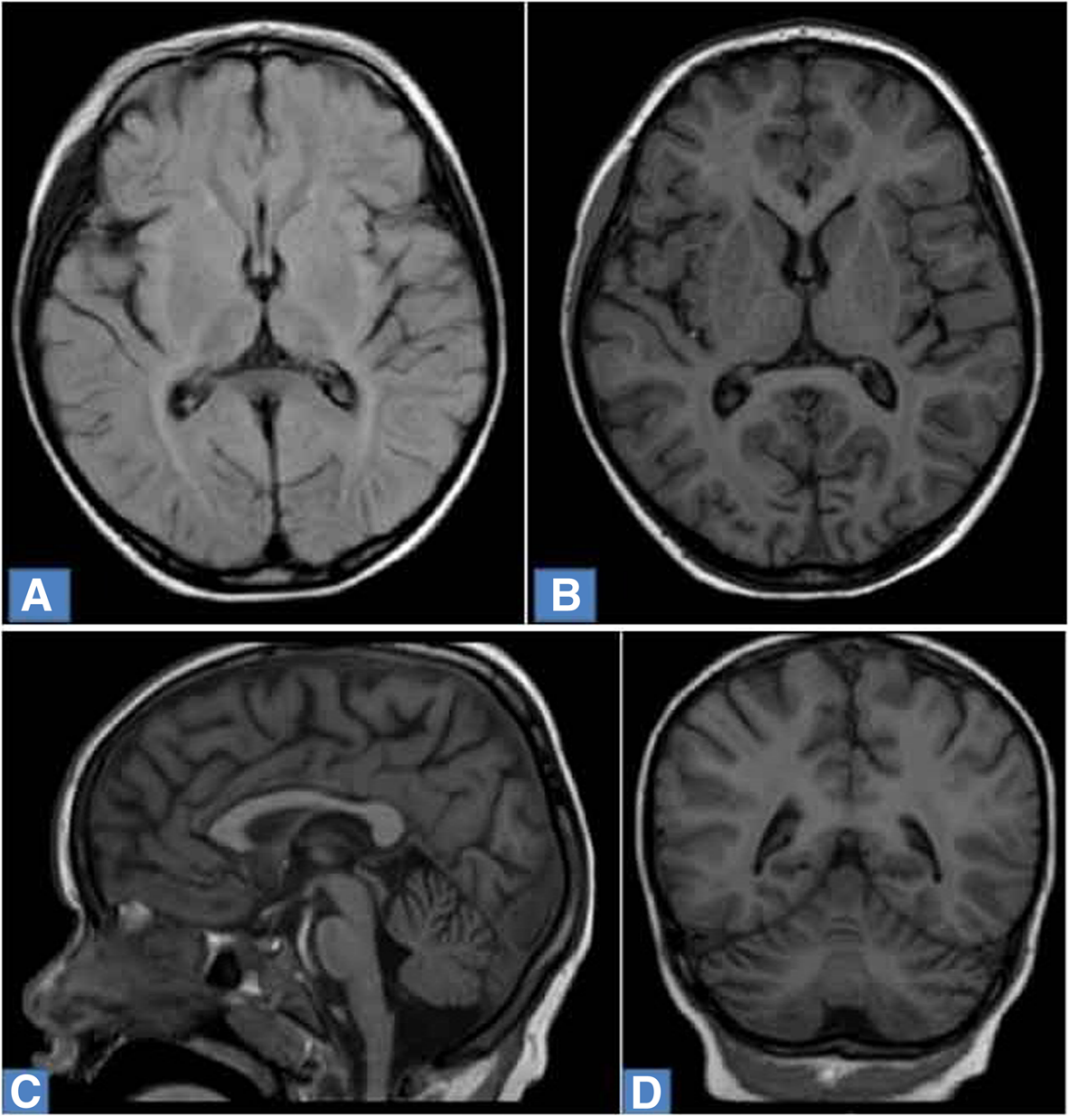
Brain MRI of 5-year-old girl with NCL7. a. Diffuse cortical atrophy, increased intensity of the MR signal in periventricular white matter. Axial T2 weighted FLAIR image. b. Diffuse cortical and subcortical atrophy of the brain. Axial T1 weighted image. c. Atrophy of the cerebellum, cortical atrophy of the brain. Sagittal T1 weighted image. d. Diffuse cortical and subcortical atrophy of the brain, atrophy of the cerebellum. Frontal T1 weighted image
